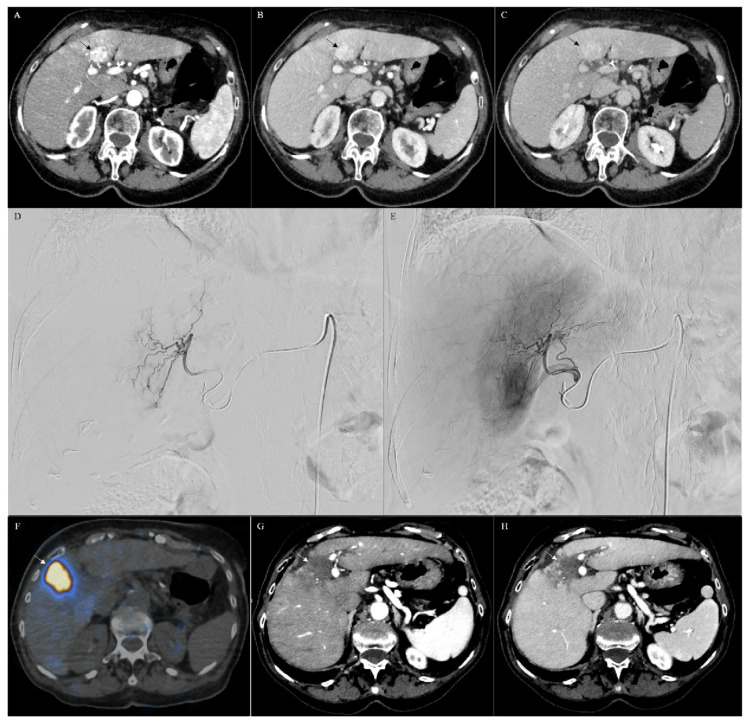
CT of a combined hepatocellular cholangiocarcinoma (arrow) (A–C). In particular, CT showed a lesion in the segment IV of the liver with a strong hyperenhancement during the arterial phase (A), followed by a persistent enhancement during the portal (B) and delayed phases (C), consistent with the imaging diagnosis of combined hepatocellular cholangiocarcinoma, thereafter confirmed with biopsy. The lesion was treated by using transarterial radioembolization (TARE). In particular, during the angiographic study (D,E), the artery feeding the liver segment IV was selectively catheterized (D), the lesion was confirmed with selective angiography (E), and the intra-arterial treatment was selectively performed. Positron emission/computerized tomography (PET/CT (F)) demonstrated that the treatment was correctly performed, covering all the lesion (arrow in (F)). CT scan, performed 6 months after TARE, demonstrated complete response of the lesion characterized by no vascularized area in the IV segment of the liver in the arterial (arrow in (G)) and in the venosus (arrow in (H)) phases.
